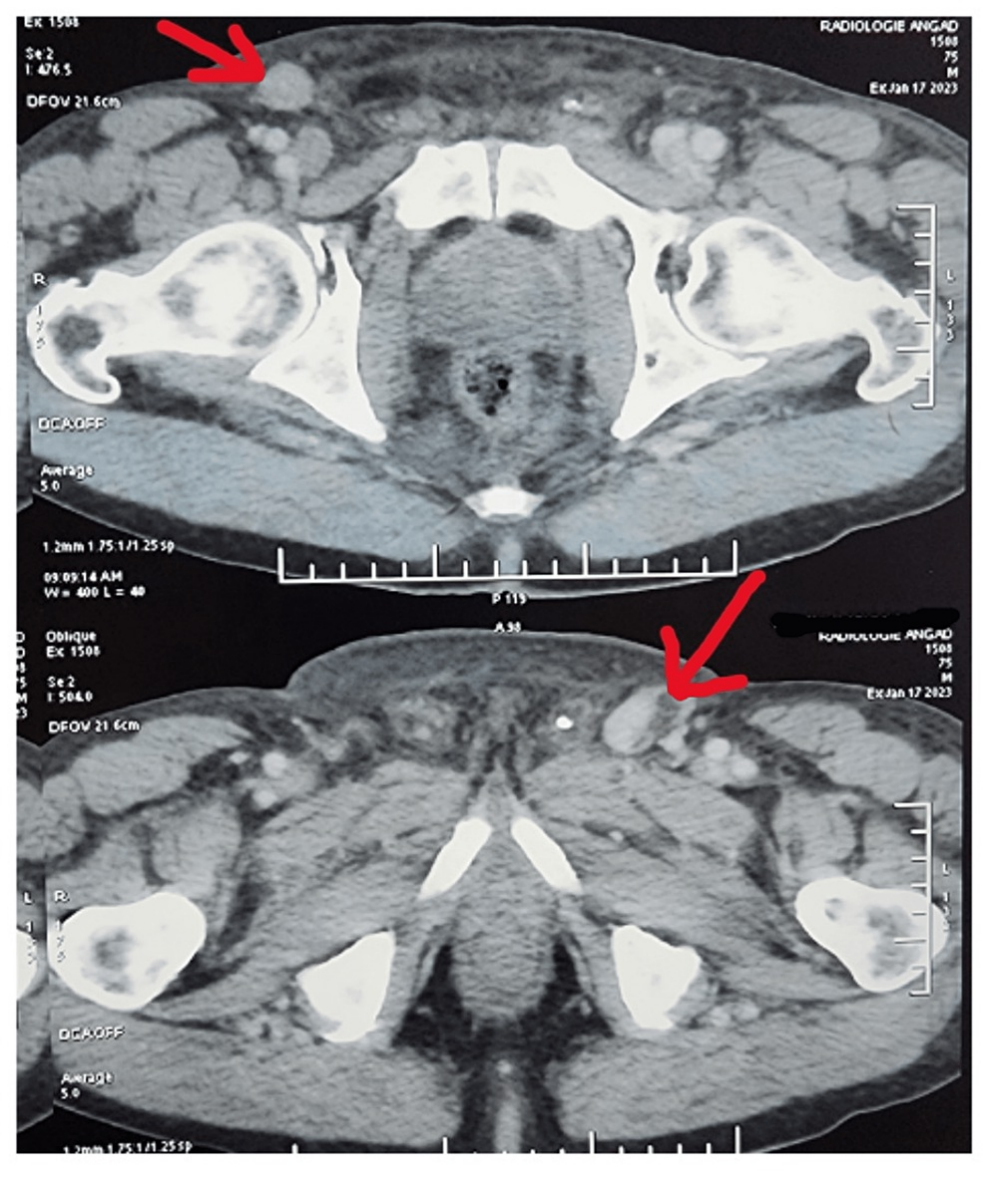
CT scan of the thorax, abdomen, and pelvis revealed bilateral inguinal lymphadenopathies.
